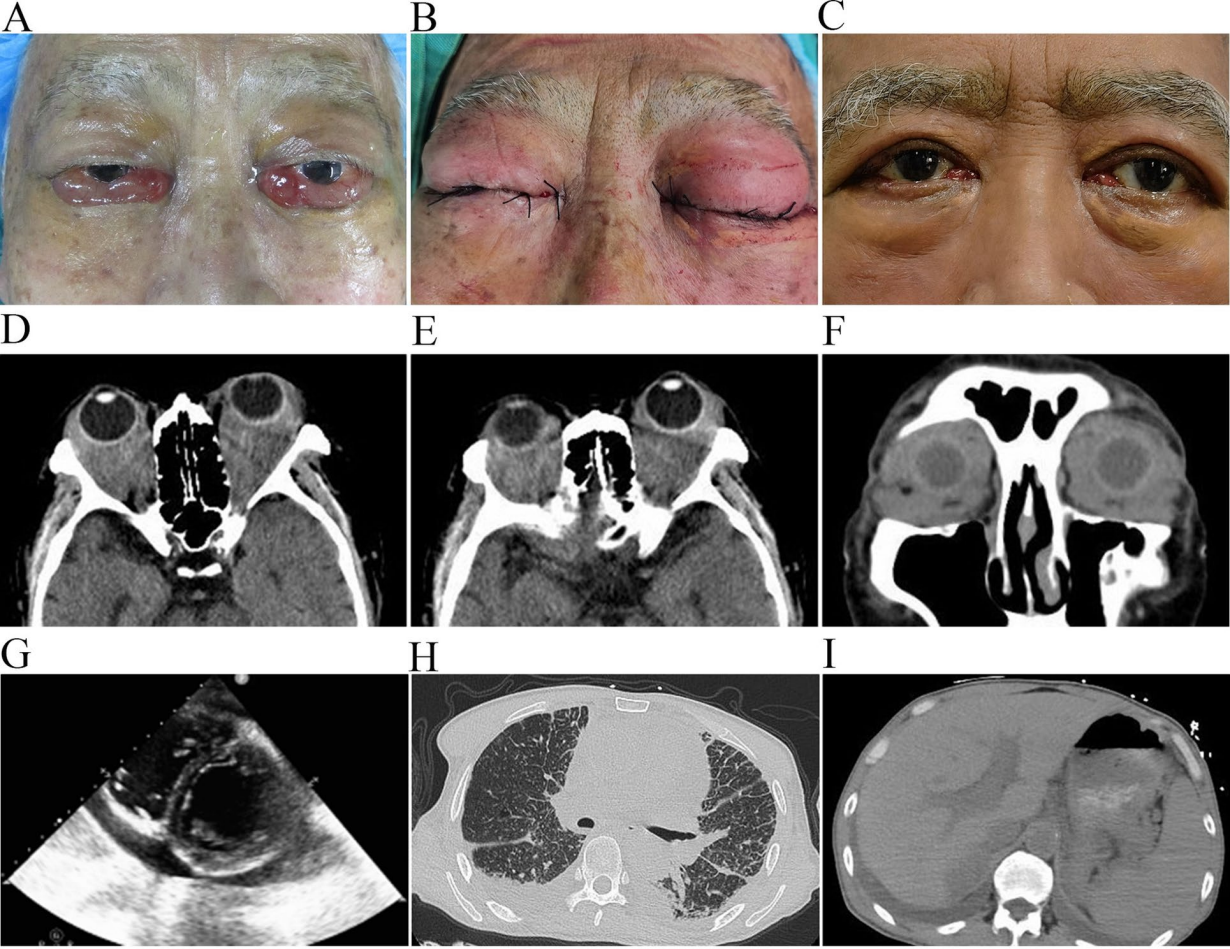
A Proptosis, bulbar conjunctival congestion, and edema of both eyes. Xanthelasma was noted around both eyes. B Eyelid swelling postoperatively. C After treatment with vemurafenib, the patient returned to normal overall, but vision recovery was impossible. D–F Orbital CT. A soft tissue density shadow can be seen in the bilateral orbit, which partially surrounds the eyeball and optic nerve. G Pericardial effusion and left ventricular hypertrophy were observed by echocardiogram. H CT showed pleural effusion. I CT showed ascites and swelling of the kidney
